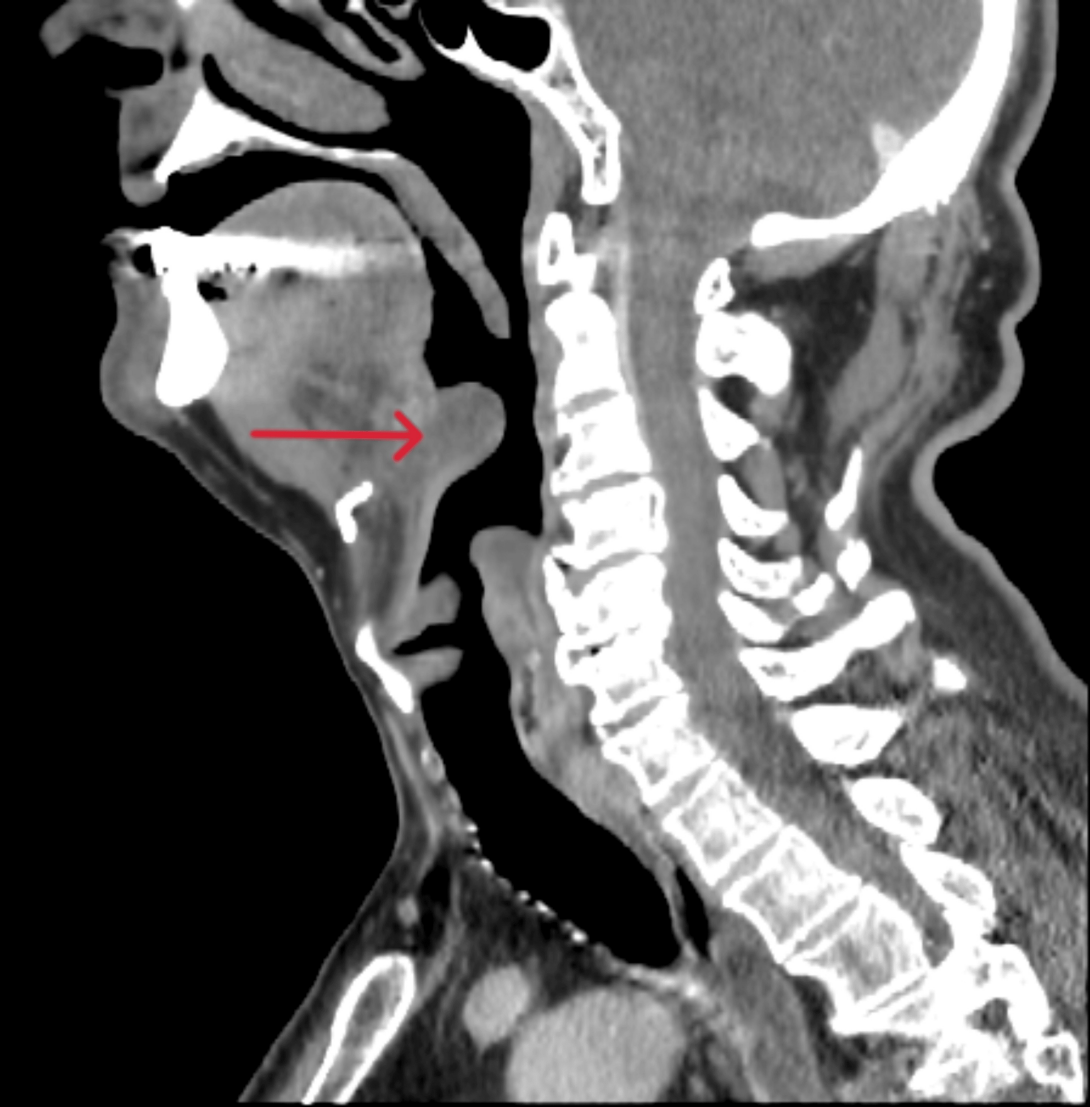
A CT scan of the neck soft tissue with intravenous contrast revealed mild to moderate laryngeal edema extending superiorly and involving the epiglottis (red arrow), concerning for epiglottitis versus iatrogenic from recent instrumentation. There was no evidence of airway stenosis.
